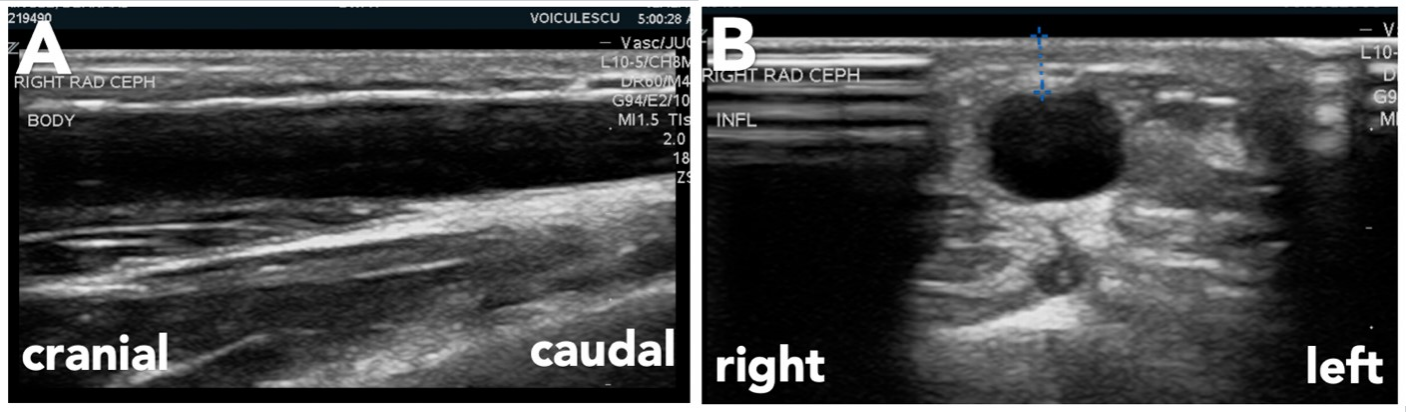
Ultrasound planes: A) longitudinal axis; B) transverse axis. Figures courtesy of Dr. AdinaVoiculescu.
